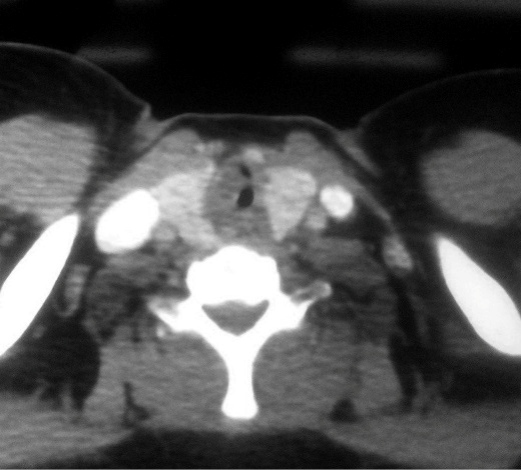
Contrast-enhanced axial computed tomography (CT) scan of neck shows a broad-based soft tissue mass arising from posterior and right side wall wall of trachea causing near total luminal narrowing and having both intraluminal and extraluminal components
